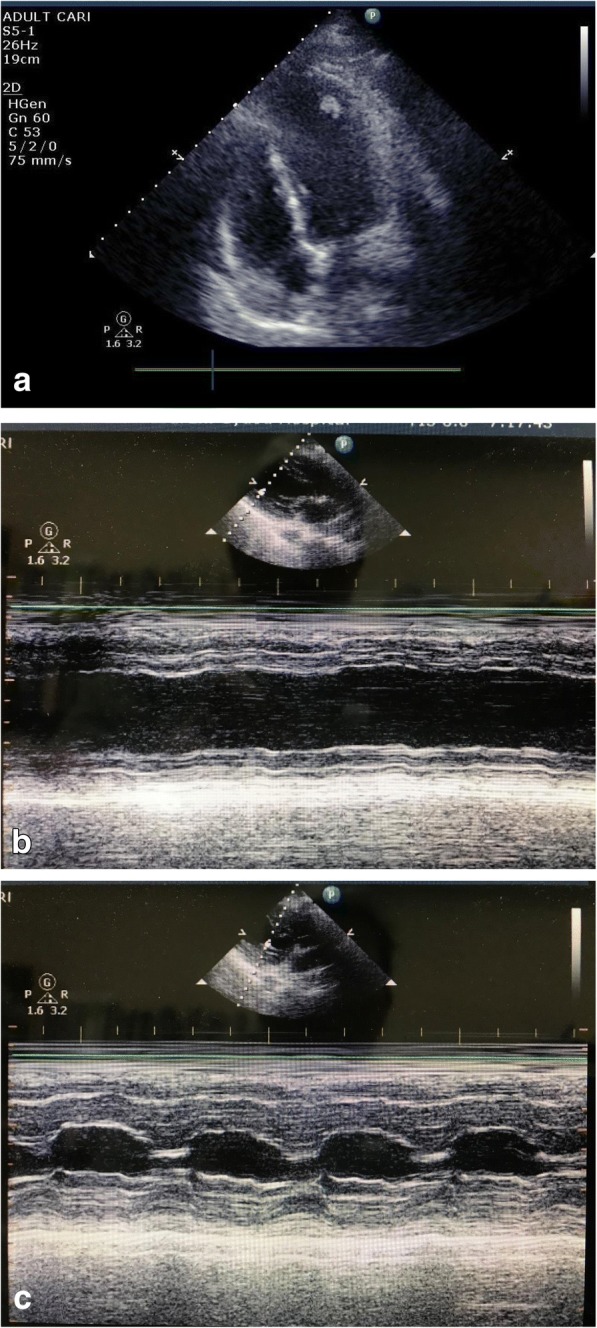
Results from the transthoracic echocardiography (TTE) performed at the ICU. A thrombus can be seen at the apex of the left ventricle. a Left ventricular thrombus. b Severe hypokinesis in apical segments. c Preserved contractile in basal segments
